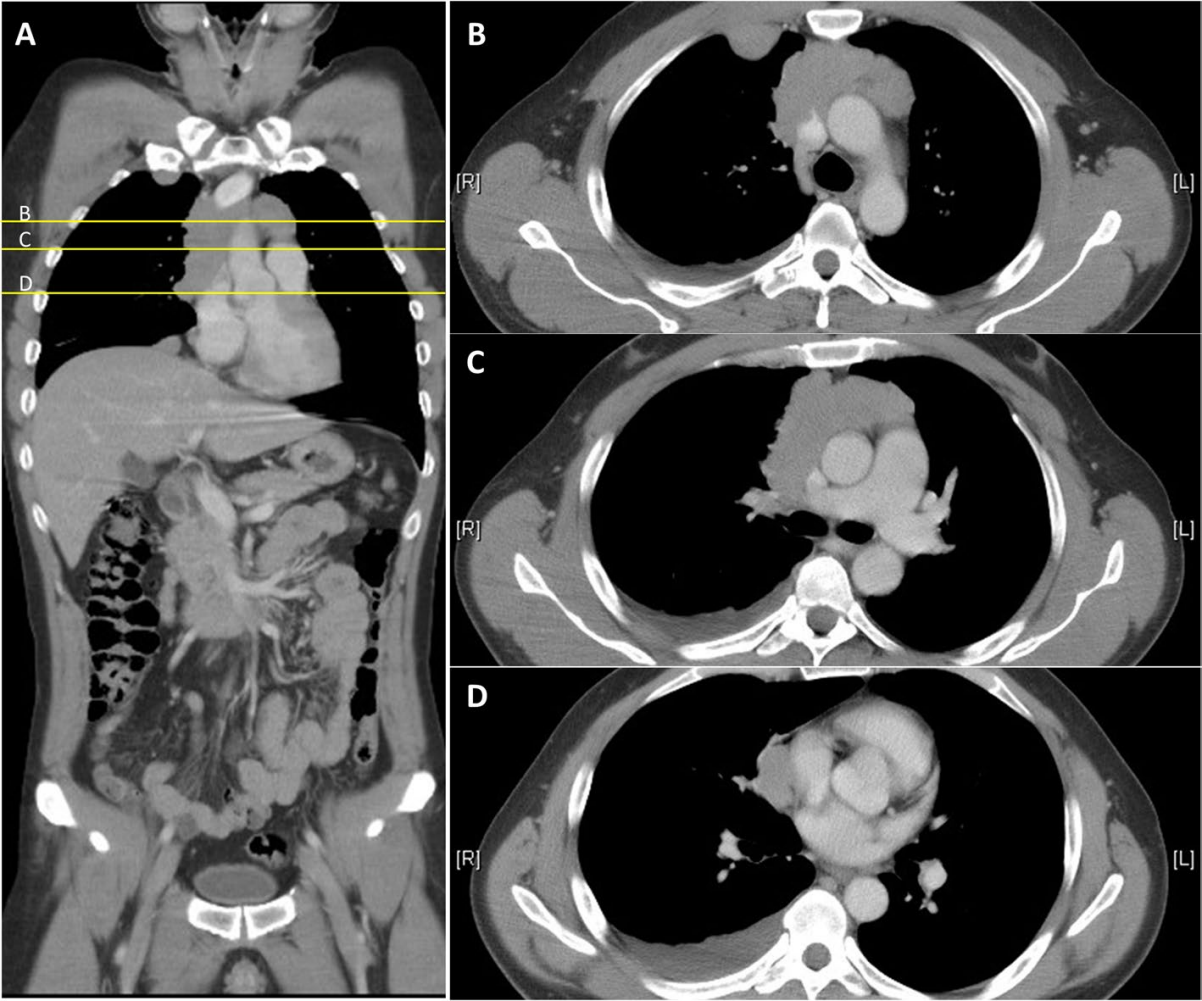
Whole-body computed tomography (CT) image. The coronal whole-body CT study of this patients (A) showed an enhancing mass lesion (8.7 × 3.9 cm) at the anterior mediastinum, which is suspected as a thymoma, with tumor attachment/invasion of ascending aorta, pulmonary artery, and partial encasement of superior vena cava (B, C). Nodular thickening of the right pleura (B) and right-side pericardium (D), which are suspected tumor invasions are also noted
